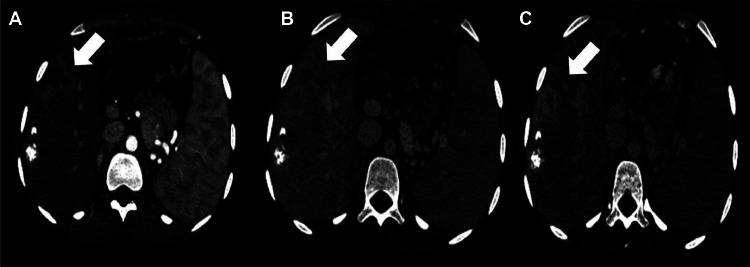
Axial CT scan showing a residual hypervascular tumor in segments VI–VII (A); reappearance of enhancement suggestive of viable tumor tissue.
